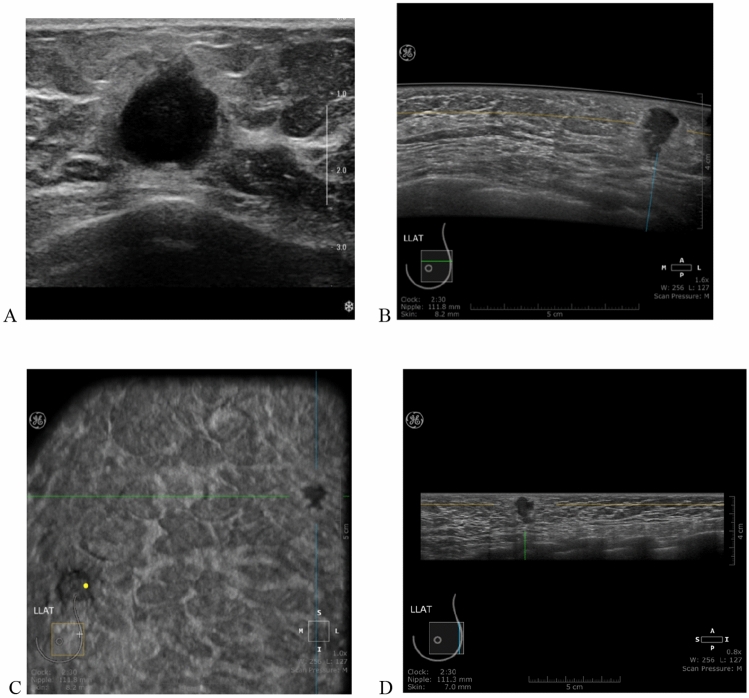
A 32-year-old woman with ductal carcinoma of the left breast. (A) shows a hypoechoic lesion with handheld ultrasound (HHUS). And (B,C,D) show the heterogeneous hypoechoic lesions in the medial (B), lateral (D), and anterior–posterior (C) position of Automated Breast Ultrasound (ABUS).
